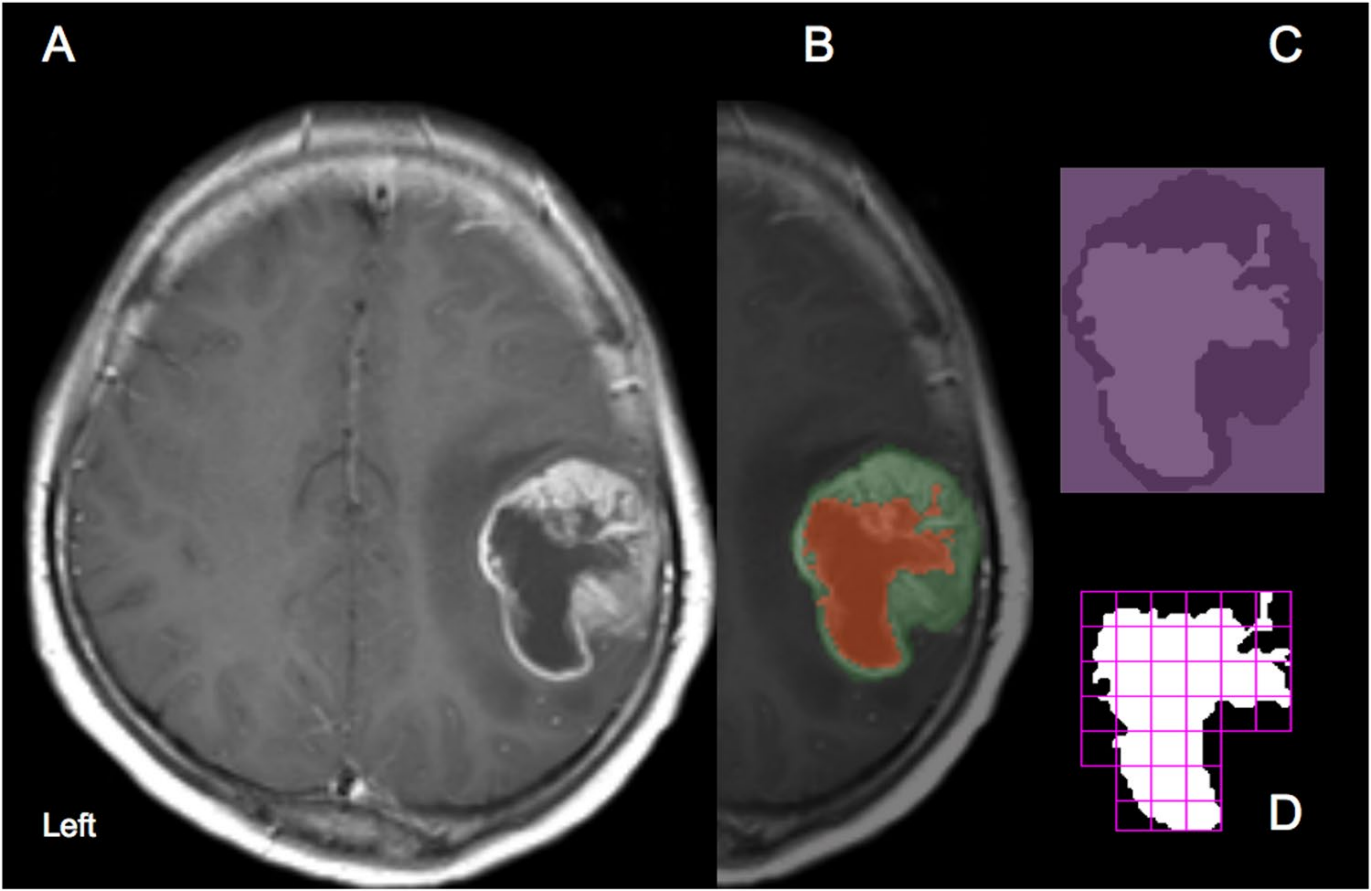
Segmentation and fractal analysis procedures for the analysis of necrotic patterns in glioblastoma. (A) A post-contrast T1-weighted magnetic resonance image of a representative patient with glioblastoma. (B) Segmentation of the tumor (green mask) and necrotic ROIs (red mask). (C) The necrotic ROIs are extracted with the tumor ROIs as the border. (D) Fractal analysis was performed by using the box-counting method.
